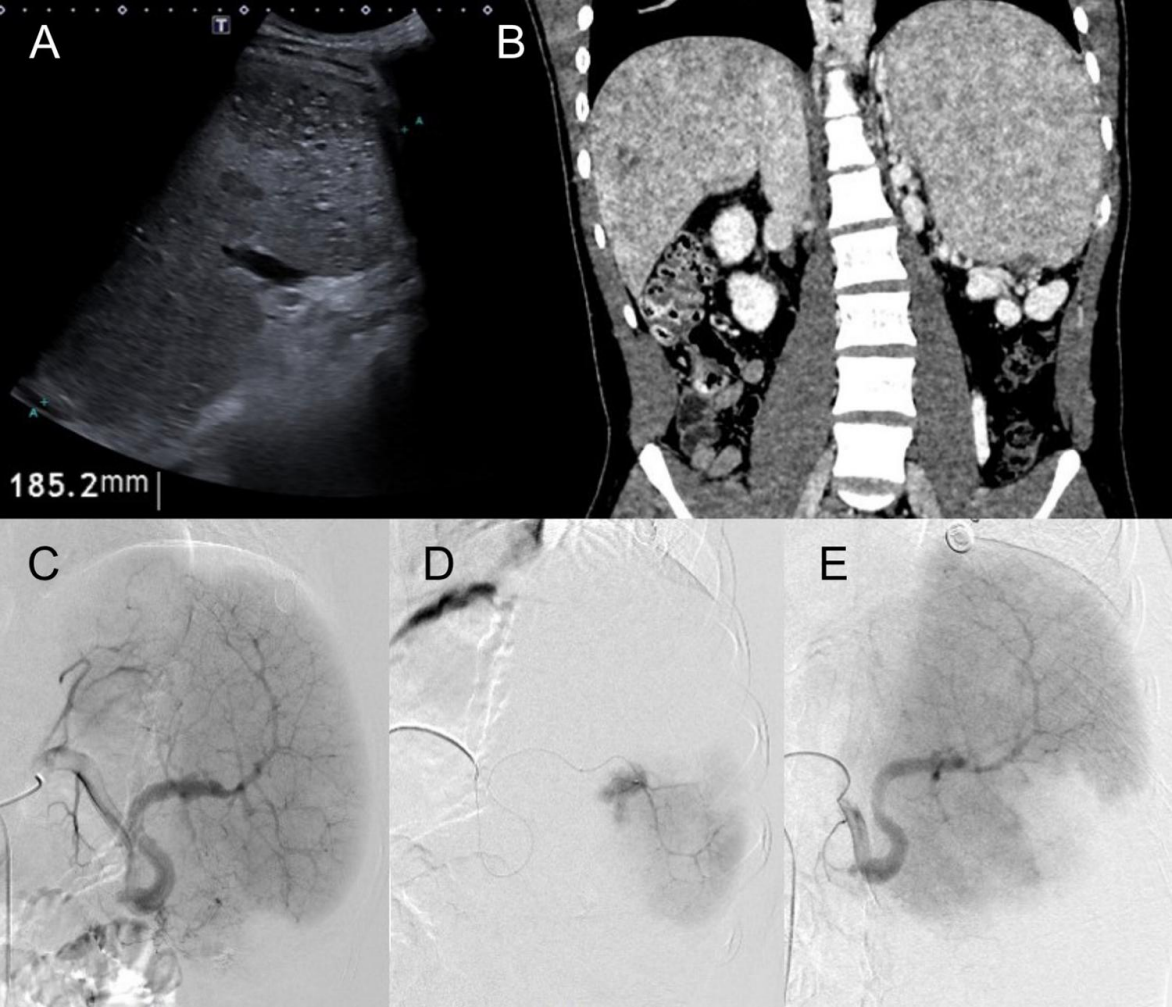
(a) Total abdominal ultrasound and (b) abdominal angiotomography in multiplanar reconstruction in coronal section in portal phase showing splenomegaly. (c) Angiography of the spleen from the splenic artery. (d) Superselective angiography in the middle third of the spleen showing healthy parenchyma. (e) Absence of contrast in the middle third of the spleen demonstrating its embolization.
